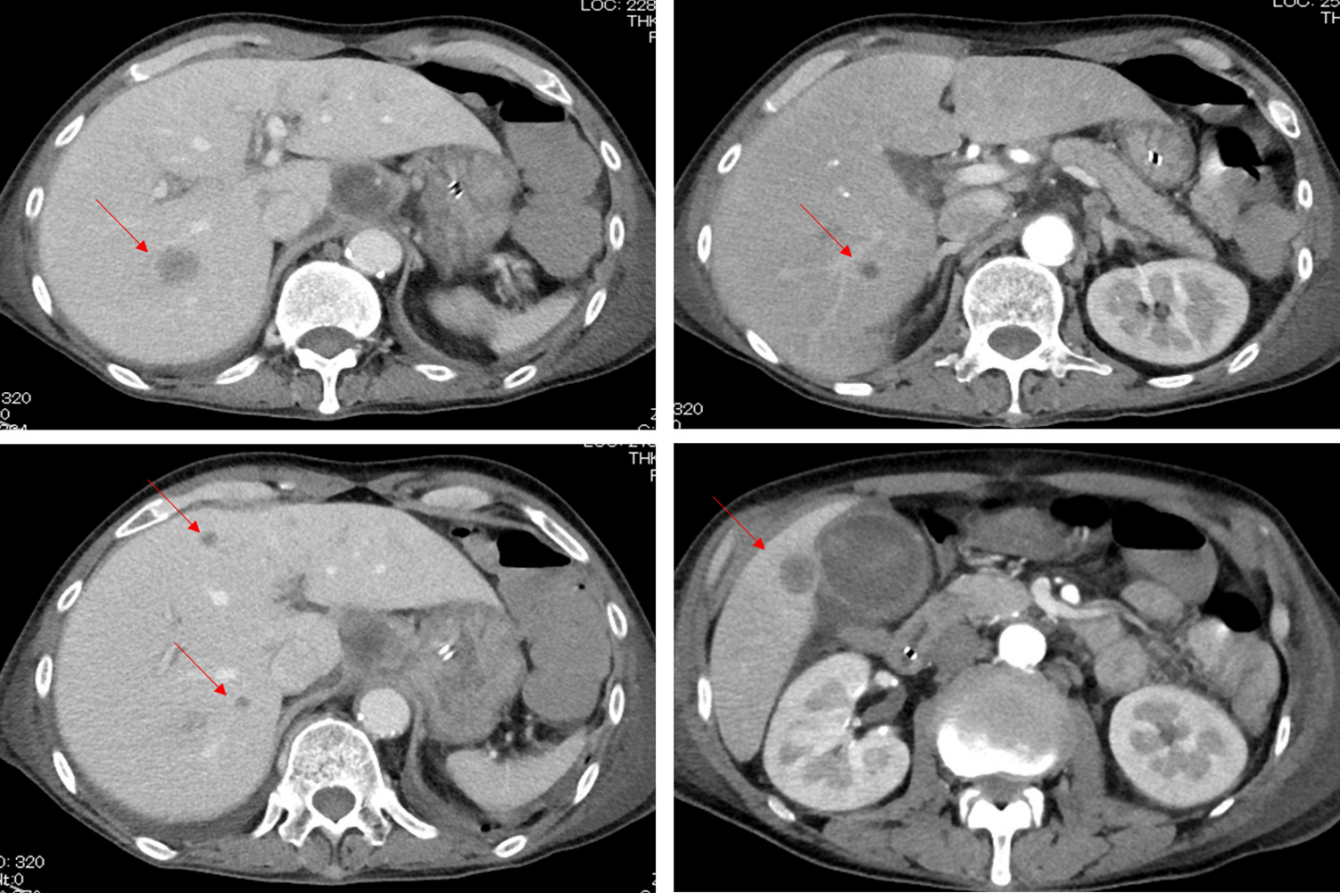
Contrast CT imaging. The arrows indicate the metastatic liver lesion.
